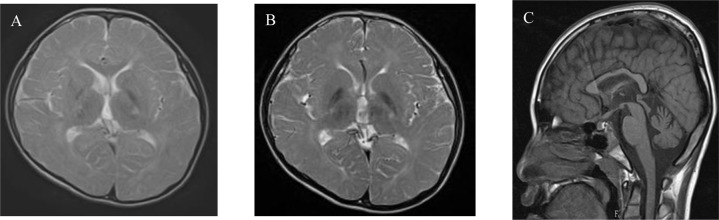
Brain MRI images. A Axial T2 image at the age of 8 years shows diffuse hypomyelination without atrophy of the basal ganglia. B Axial T2 image at the age of 11 years shows persistent hypomyelination without atrophy of the basal ganglia. C Sagittal T1 image at the age of 11 years shows atrophy of the cerebellum.
